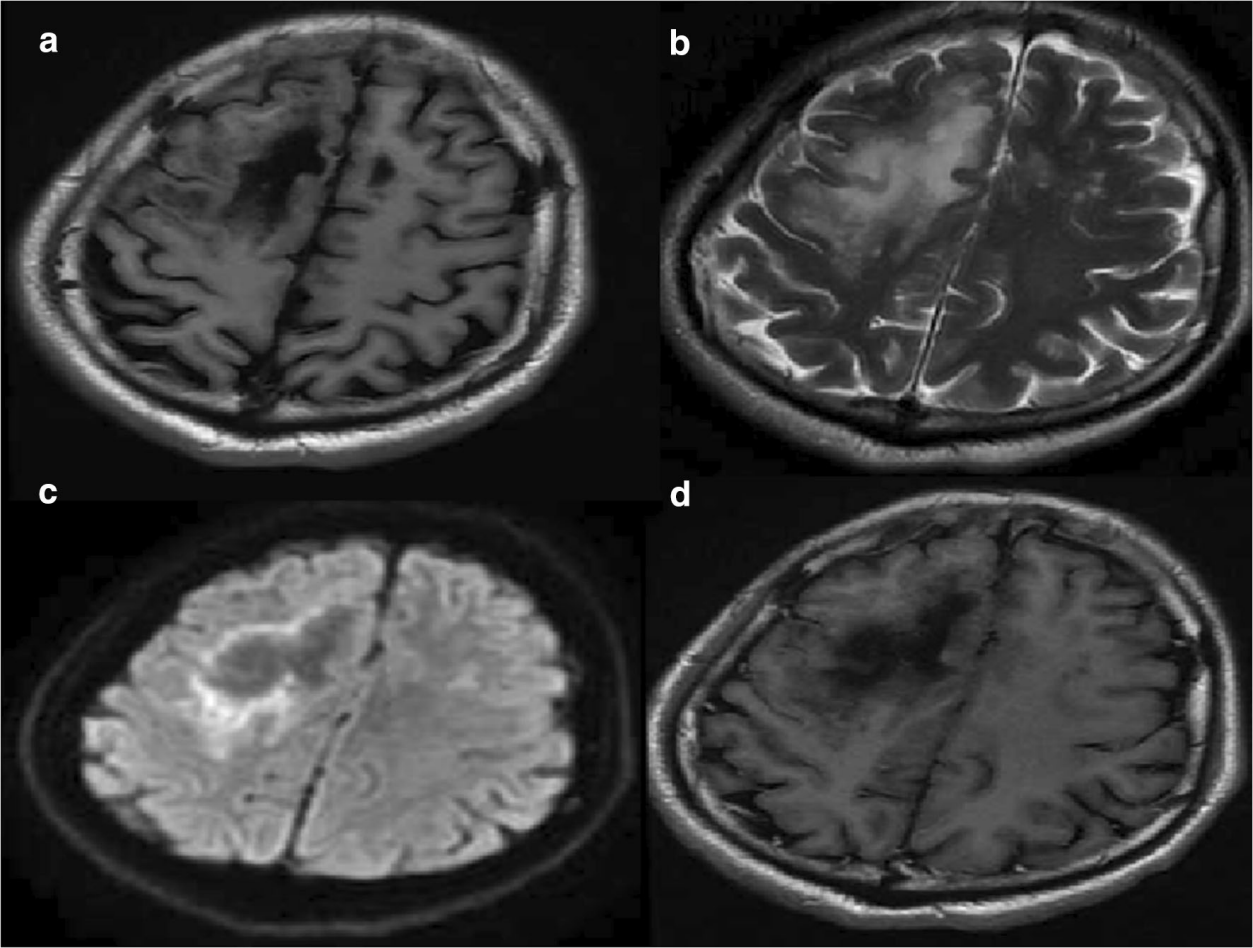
Magnetic resonance imaging results. T1-weighted images (a) showed patch-shaped signal shadows on the right frontal parietal occipital lobe and left frontal-temporal lobe. These lesions were hyperintense on T2-weighted images (b). Diffusion-weighted images (DWI) demonstrated ring-shaped marginal and small patch-like high signal shadows (c). No significant enhancement was seen after enhancement (d)
